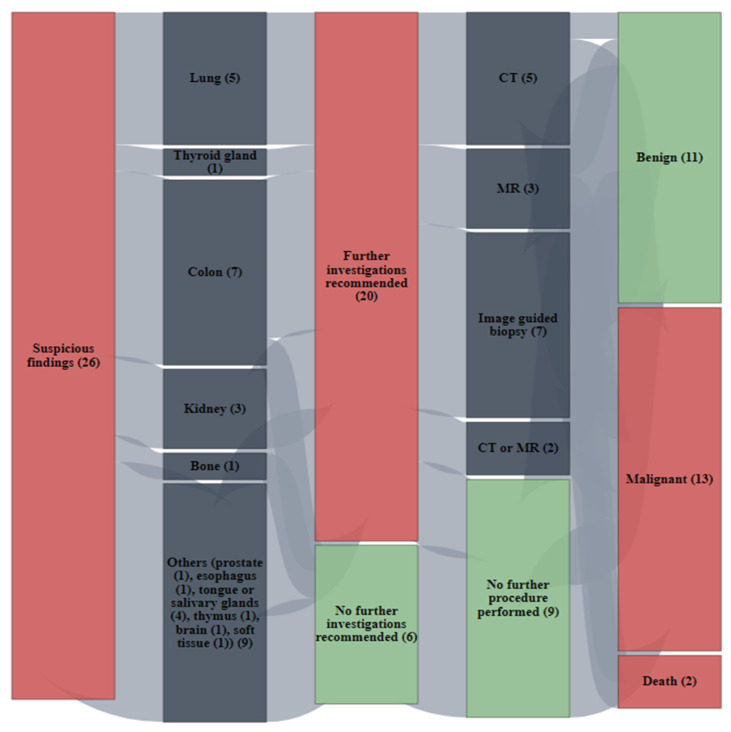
Sankey plots display the number of suspicious findings (total and per organ) detected incidentally on FDG-PET/CT in NSCLC patients at staging. It is also displayed, whether further investigations into the suspicious findings were recommended or not by the reporting physicians, as much as the nature of the findings after examinations.
